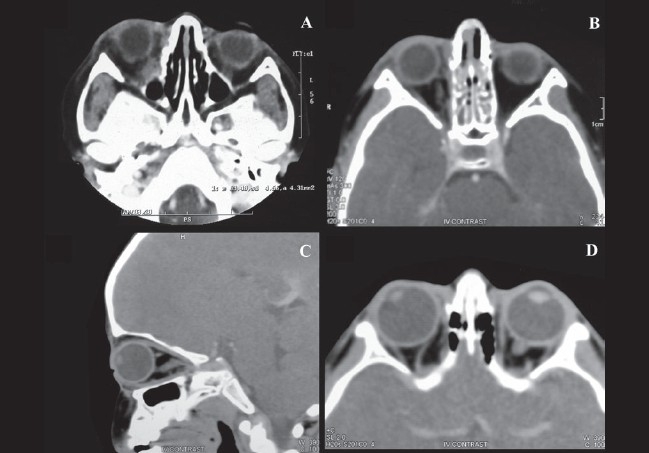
(A) CT scan post chemotherapy suggestive of reduction in mass infero-medially and posteriorly in the right orbital apex. (B) CT scan post radiotherapy and chemotherapy with further reduction of tumor mass. (C and D) CT scan on the last follow-up (30 months) shows no recurrence or residual disease
